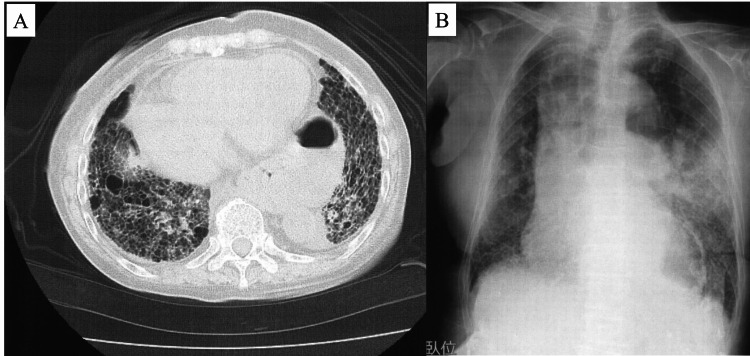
X day (admission) Chest CT results showed an exacerbation of pulmonary interstitial shadows, mainly frosted shadows (A). The X-ray images showed frosted shadows in the middle and lower lobes (B).
